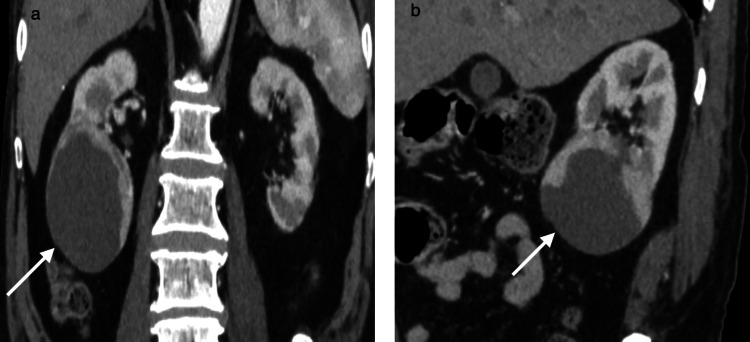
(a) Coronal contrast-enhanced CT (CECT) (corticomedullary phase) shows a 6.8 × 6.6 × 6.4 cm exophytic cystic lesion from the right lower pole abutting the inferior calyx. A 13 mm mural nodule enhances from 12 HU (pre) to 35 HU (post), consistent with Bosniak IV. No septations were noted. (b) Sagittal CECT confirms an exophytic lesion with an enhancing mural nodule (white arrow, 13 mm, 35 HU post-contrast). The lesion abuts the inferior polar artery without invasion.
